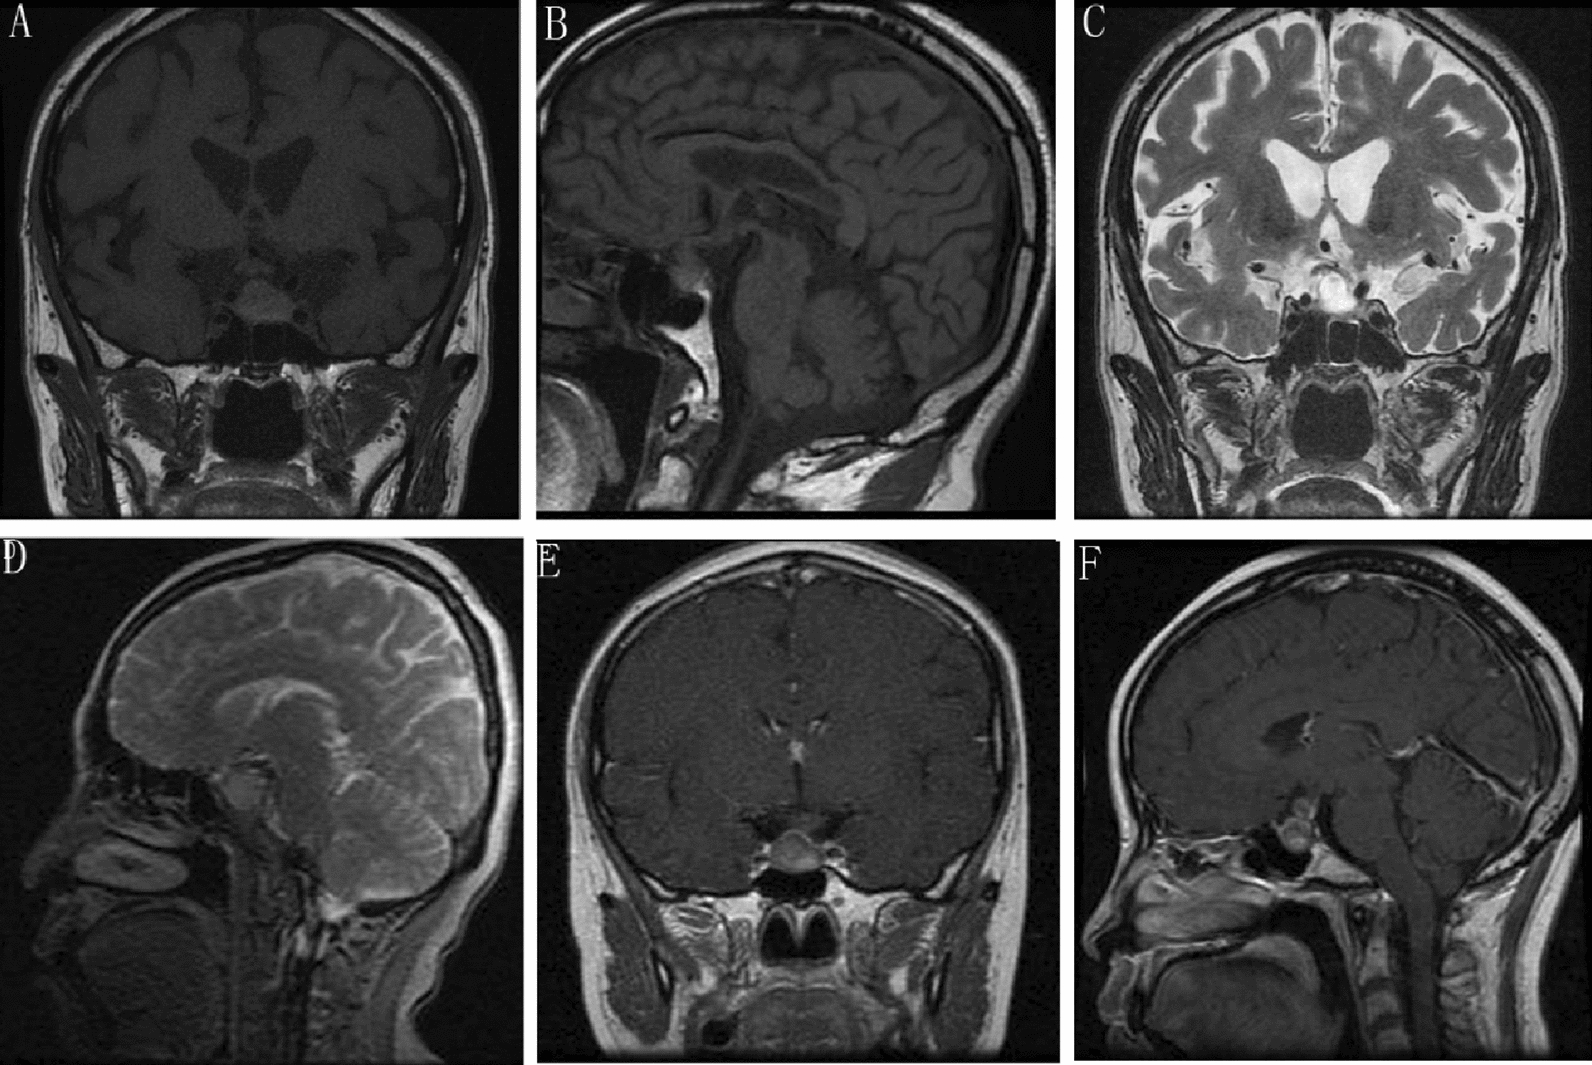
MRI of PA preoperative: A T1WI coronal: iso-hyperintense signal; B T1WI sagittal: iso-hyperintense signal; C T2WI coronal: hyperintense signal revealed a sellar heterogeneous; D T2WI sagittal: hyperintense signal; E T1WI coronal contrast-enhanced: heterogeneous hyperintense signal, lesion with rim enhancement; F T1WI sagittal contrast-enhanced: heterogeneous hyperintense signal, lesion with enhancement
